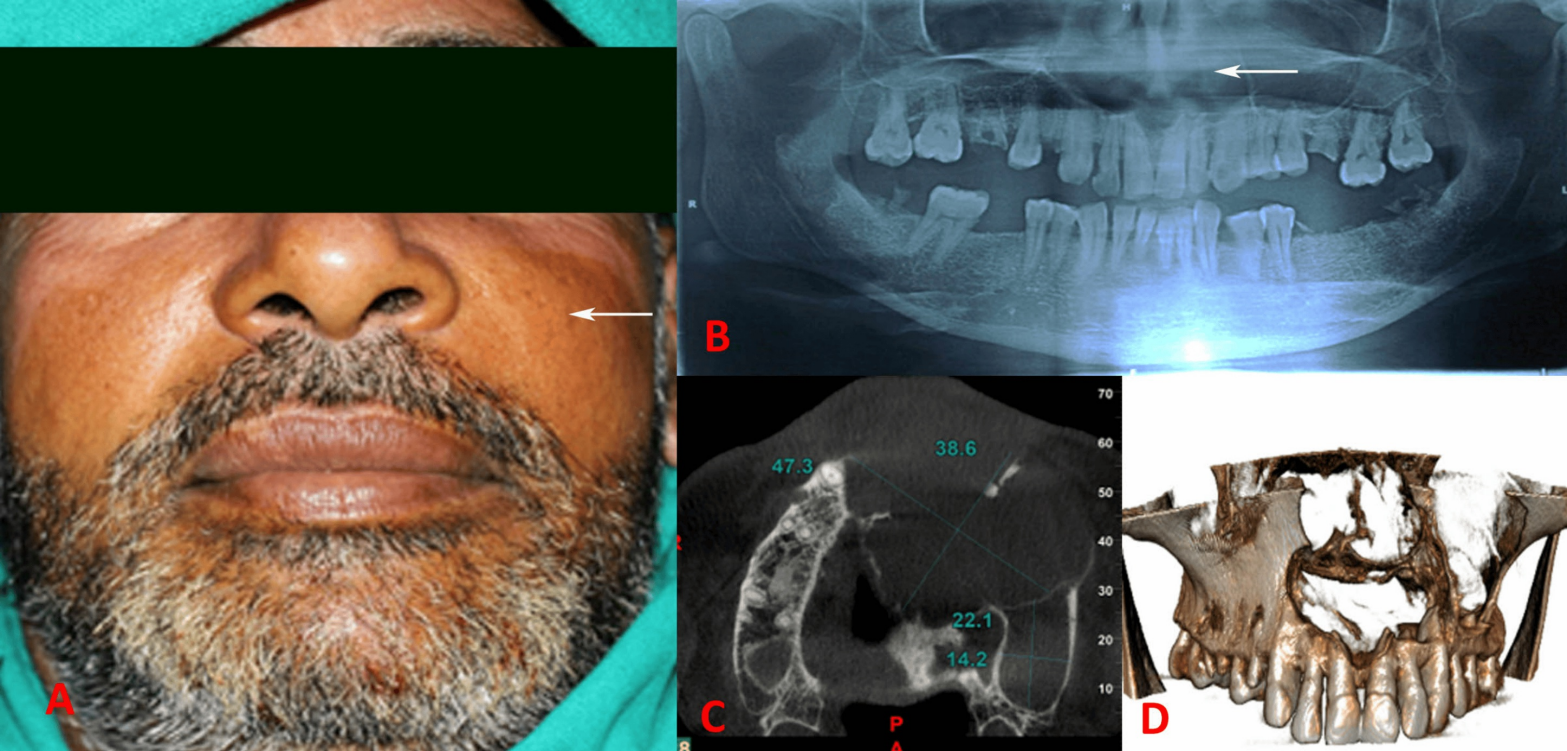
(A) Diffuse swelling on the left maxilla obliterating nasolabial fold, (B) OPG showing radiolucency extending from the left first molar to the anterior maxilla and involving the left maxillary sinus, (C) size of the cystic radiolucency, (D) CBCT showing extension of the cyst margins.
